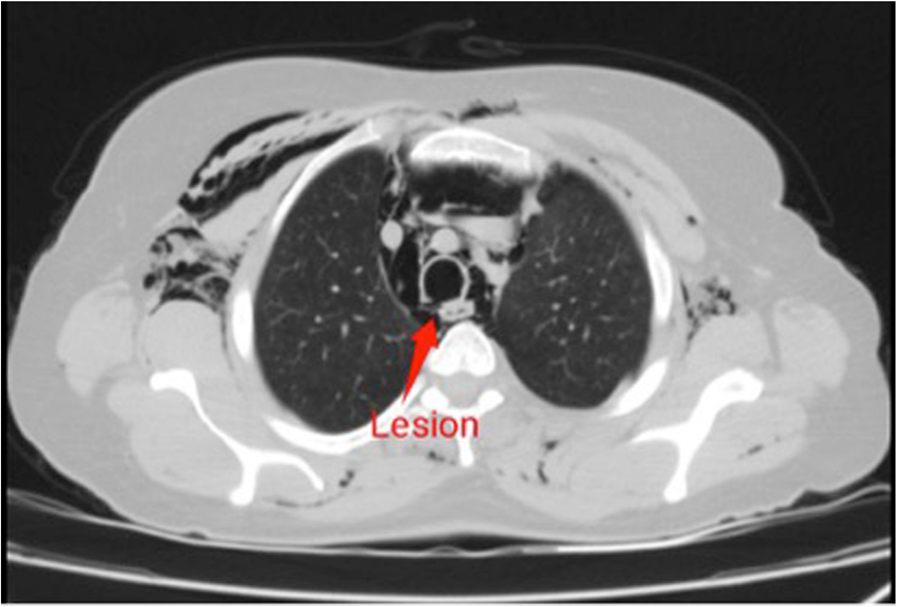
CT scan case 1 tracheal rupture
